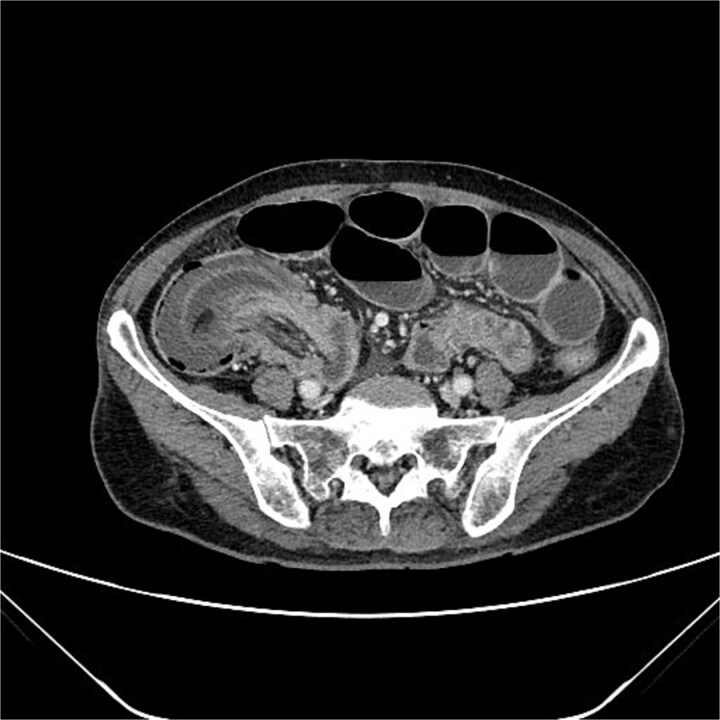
Axial abdominal CT scan.
